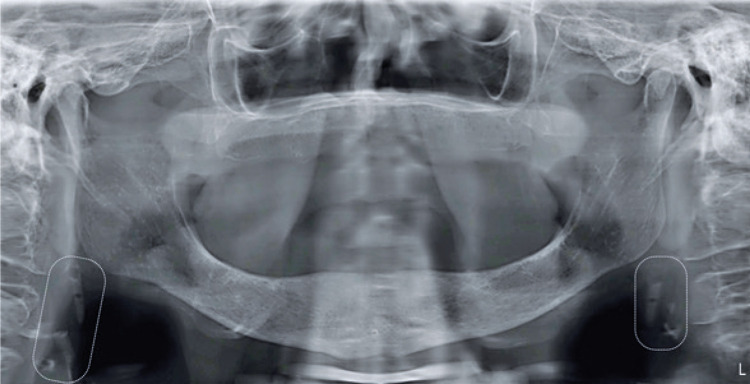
Panoramic radiography presenting areas of calcifications in soft tissue at level of vertebrae C3 and C4, bilaterally
